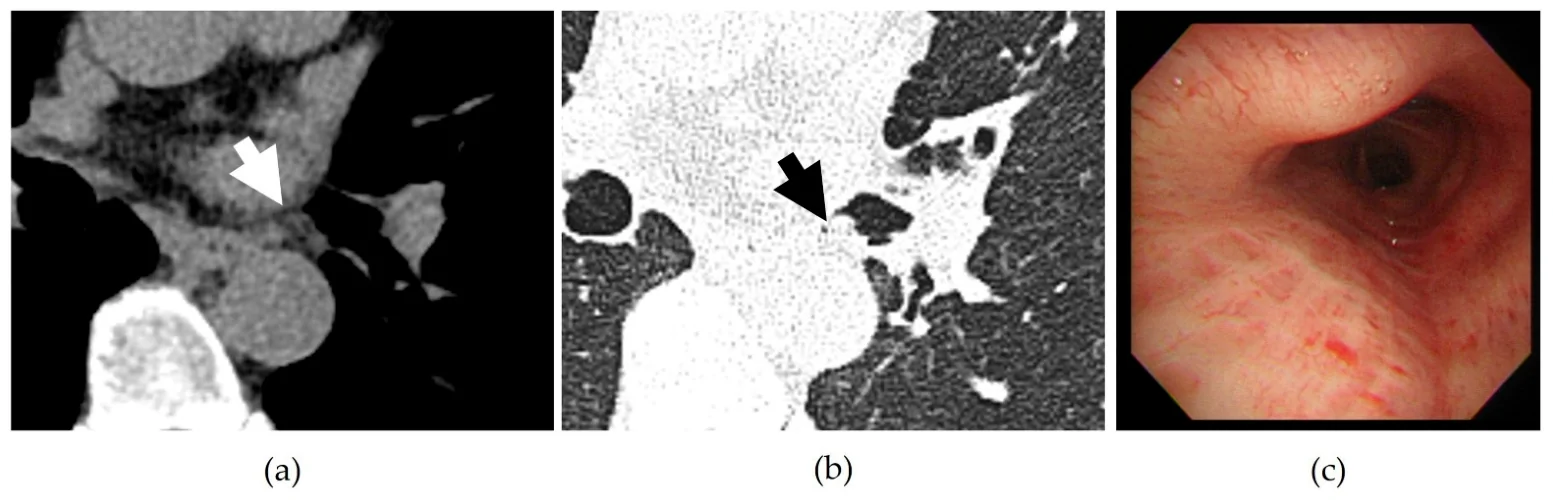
Chest CT images of a 63-year-old man with a 40-pack-year smoking history. (a) The mediastinal window CT image shows an oval-shaped, low-density lesion (arrow) in the left main bronchus. (b) Axial CT image in lung window setting with 1 mm slice thickness reveals an internal micro air bubble (arrow) within the lesion. A non-tertiary hospital radiologist categorized the lesion as Lung-RADS category 4A (suggestive of an endobronchial nodule). However, a thoracic radiologist at a tertiary hospital categorized it as category 1 (secretion). (c) Bronchoscopy revealed no evidence of an endobronchial lesion.
